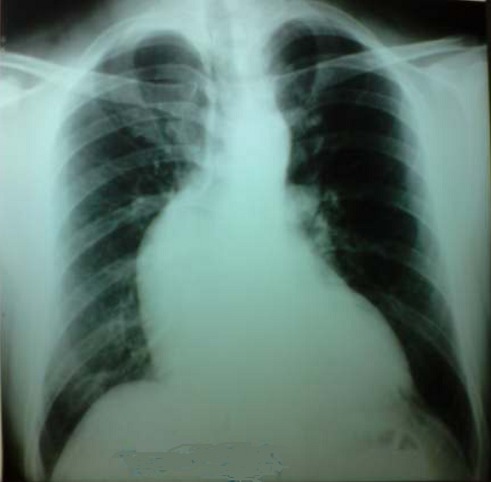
Radiographie du thorax: élargissement médiastinal
